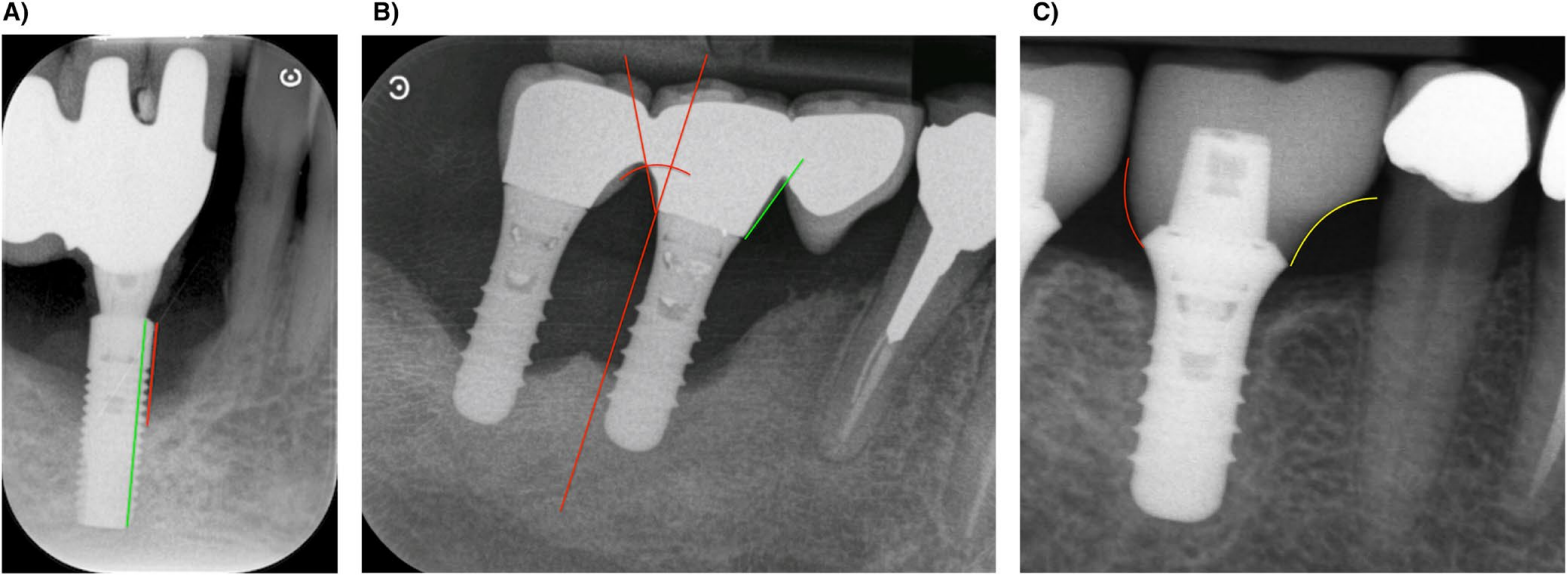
Assessment of radiographic data. (A) The bone loss was assessed in millimeters from the shoulder of the implant to the apical part of the bone defect (red line). The relative bone loss was reported as a percentage of the total implant length (green line) affected by the defect. (B) Emergence angle of the prosthetic reconstruction measured as the angle between the tangent of the transitional contour relative to the long axis of the implant (red lines). (B and C) Emergence profile of the prosthetic restauration was classified as droit (green line), convex (red line), or concave (yellow line).
